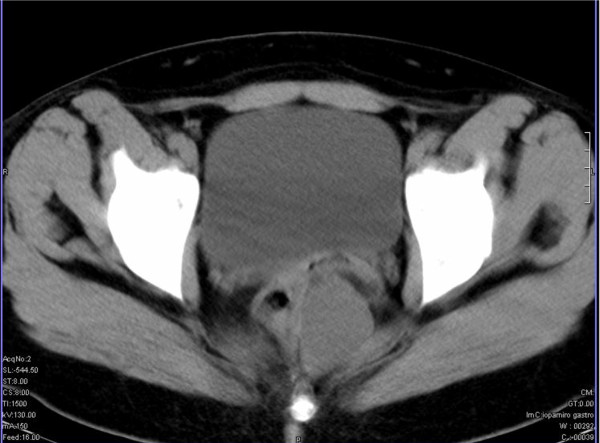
Computed tomography, showing the cystic lesion posterior and left lateral to the middle rectum.
